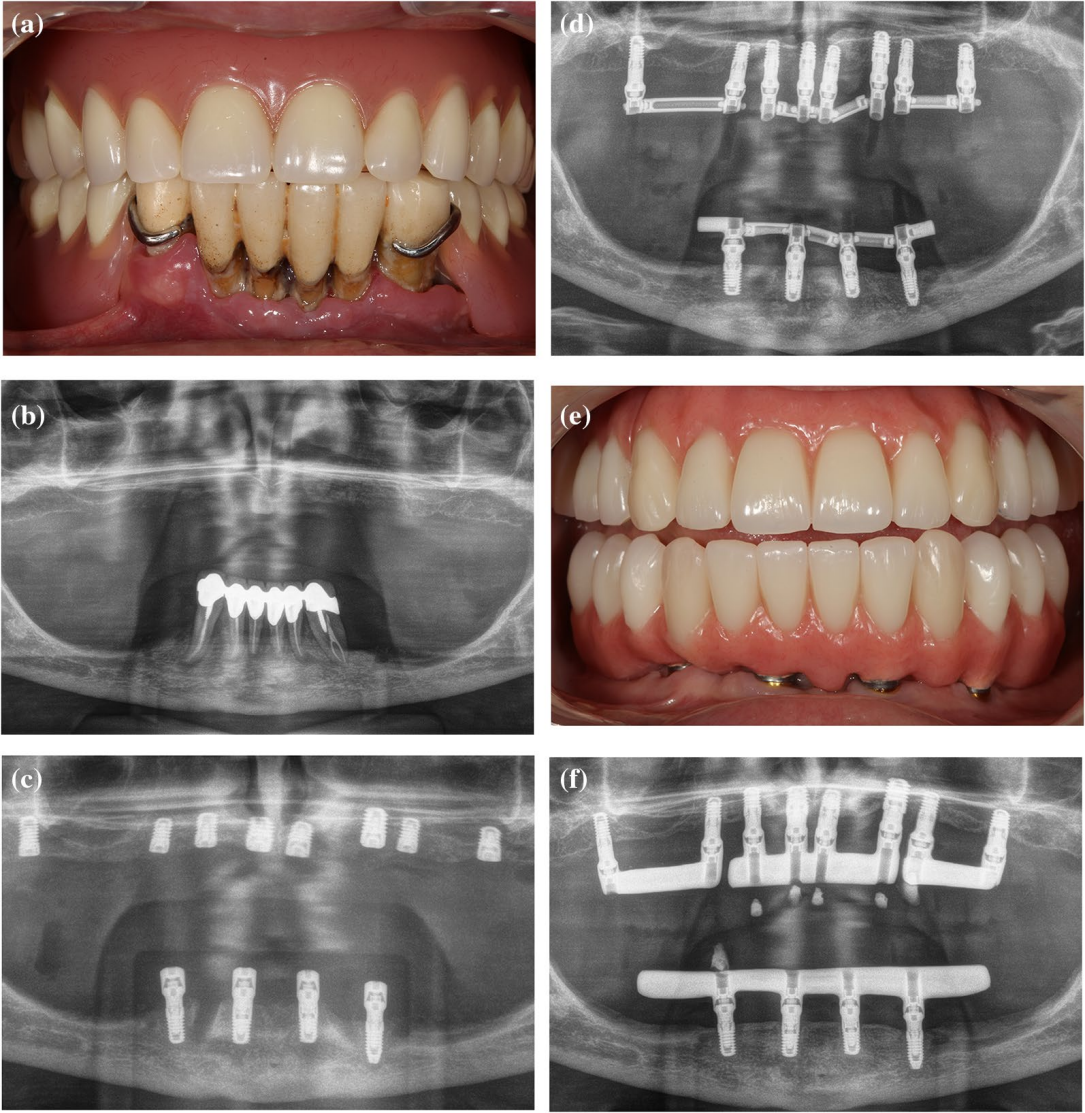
Clinical case. Full-arch maxilla rehabilitation on 8 extra-short (≤ 6.5 mm) implants. A Clinical picture showing removal complete denture in the maxilla. B Extra-oral radiograph showing the initial situation of the completely edentulous maxilla. C Placement of 8 dental implants in the maxilla. Implant diameter and length (mm): #1.1 (3.75 × 5.5), #1.3 (3.3 × 5.5), #1.4 (3 × 5.5), #1.7 (3 × 6.5), #2.1 (3.5 × 5.5), #2.3 (4 × 6.5), #2.4 (3 × 5.5) and #2.7 (3.5 × 5.5). D Provisional prosthesis screw-retained on intermediate abutments, reinforced with metal bars and resin veneered. E Clinical picture of the definitive prosthesis screw-retained on intermediate abutments. F Follow-up after 31 months of implant insertion
